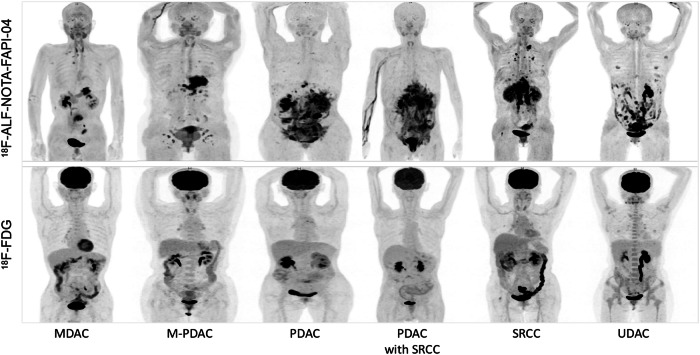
Representative MIP images of gastric cancer with various pathological types. [^18^F]ALF-NOTA-FAPI-04 PET images were superior to [^18^F]FDG PET images in terms of primary tumor and metastasis. MIP, maximum-intensity projection; MDAC, moderately differentiated adenocarcinoma; M-PDAC, moderately and poorly differentiated adenocarcinoma; PDAC, poorly differentiated adenocarcinoma; SRCC, signet ring cell carcinoma; UDAC undifferentiated adenocarcinoma
